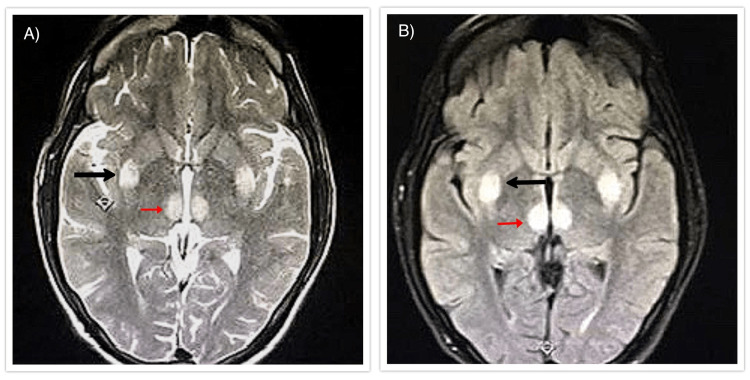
0.4 Tesla MRI demonstrates bilateral symmetric hyperintensity involving bilateral basal ganglia (black arrows) and thalami (red arrows) on axial (A) T2WI and (B) FLAIR. MRI: magnetic resonance imaging; T2WI: T2-weighted image; FLAIR: fluid-attenuated inversion recovery
